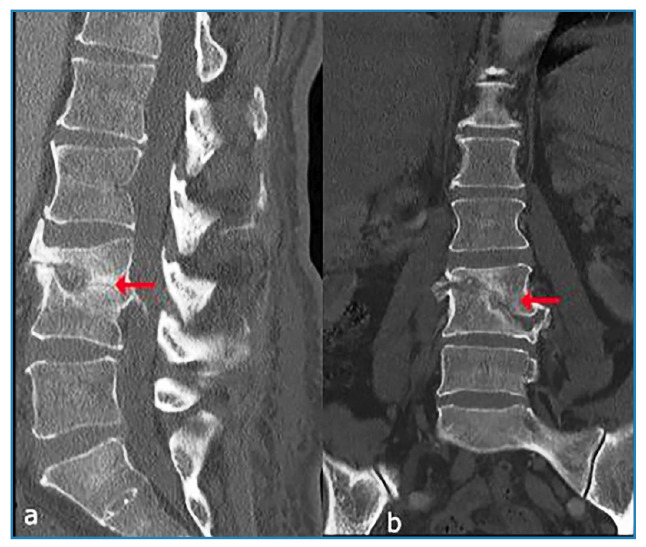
Computed tomography image of post-treatment tuberculous spondylodiscitis sequelae. (a, b) Sagittal and coronal images reveal loss of height in the L3 and L4 vertebral bodies, indicating vertebral collapse. Additionally, partial blockage of the vertebral canal is observed (arrow).
